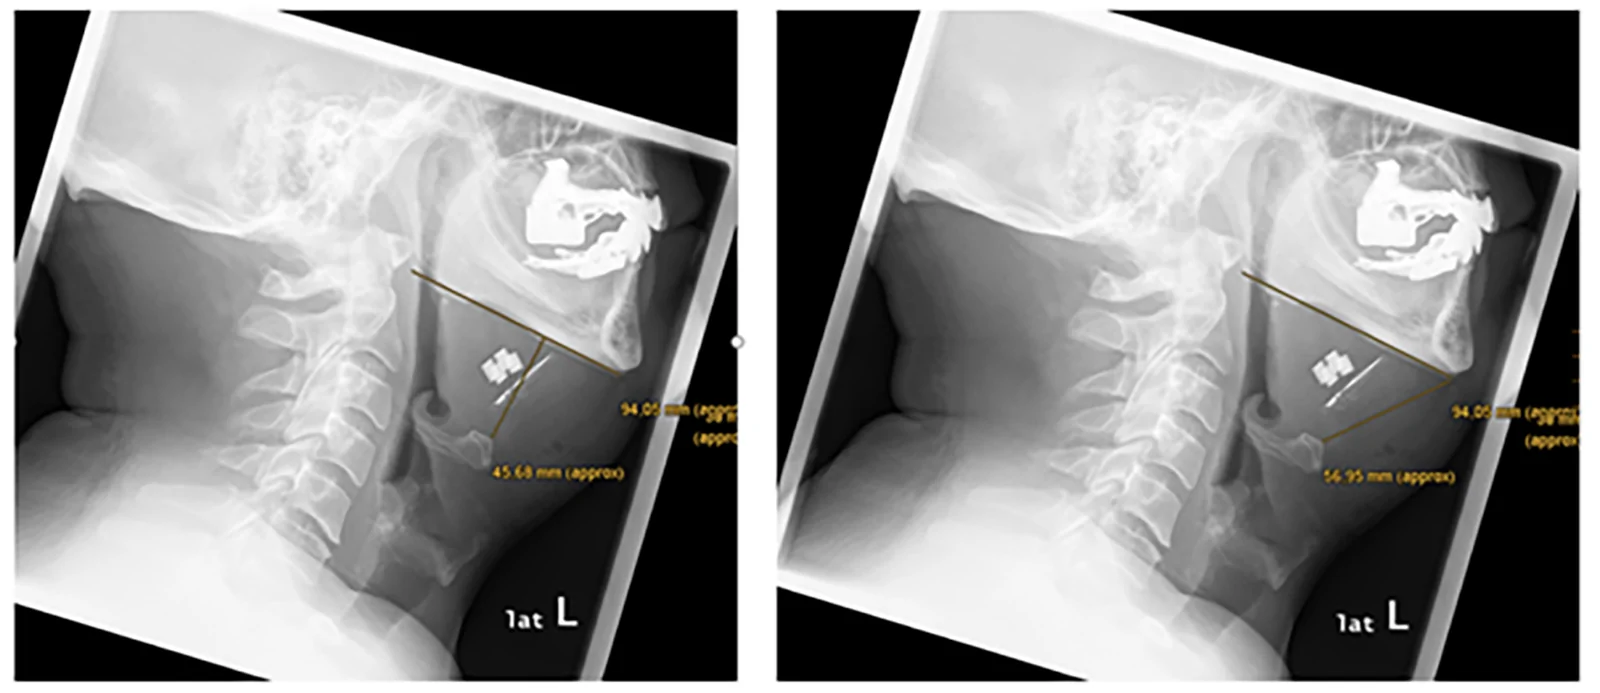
x-ray (lateral). Measurement of mandibular length (both images), distance from mandible plane to hyoid (left image), and distance from chin to hyoid bone (right image).
